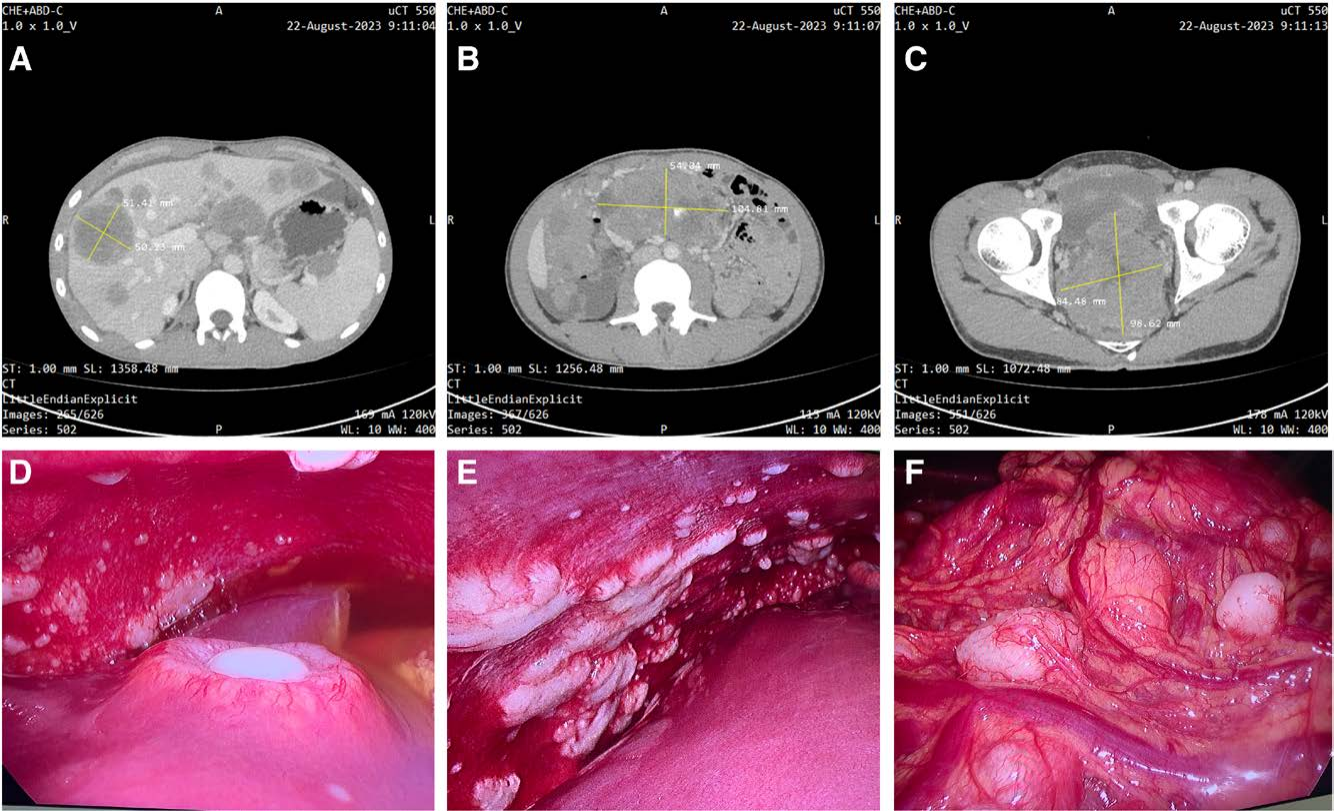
A selection of ceCT images and intraoperative observations. The ceCT revealed tumors in the abdominal and pelvic regions measuring approximately 104.81 mm × 54.04 mm and 98.62 mm × 84.48 mm, respectively. These masses displayed heterogeneous density and non-uniform enhancement. Additionally, multiple hepatic lesions with inhomogeneous density and lower enhancement were noted, the largest being about 51.41 mm × 50.23 mm in cross-section (A − C). The liver surface was irregular, featuring multiple reddish, volcano-like tumors. Additionally, numerous firm, gray-white nodules of irregular sizes, resembling grape clusters, were observed on the greater omentum and abdominal wall (D − F). ceCT = contrast-enhanced computed tomography.
